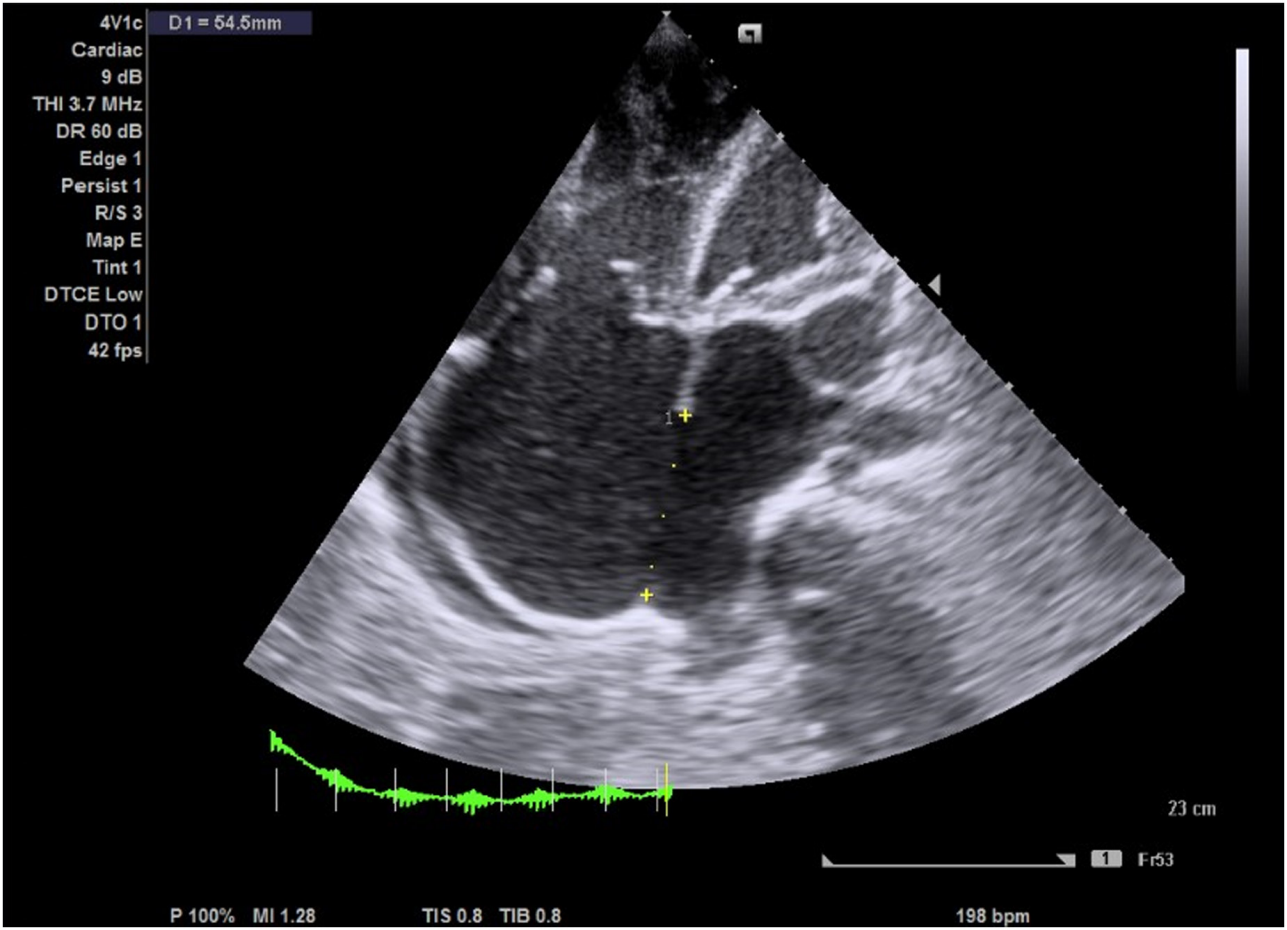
Epical 4 chamber echocardiogram showing a large atrial septal defect of size 5.45 cm and dilated right and left atria.
